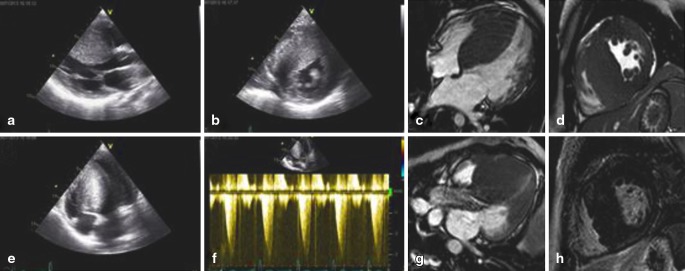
Echocardiography: parasternal long axis (a), parasternal short axis (b), apical 4-chamber view (c) and continuous wave Doppler of the outflow tract region (d), Cardiac MRI: cine image horizontal long axis view (e), short axis view (f), LVOT view (g) and LGE short axis view (h)
